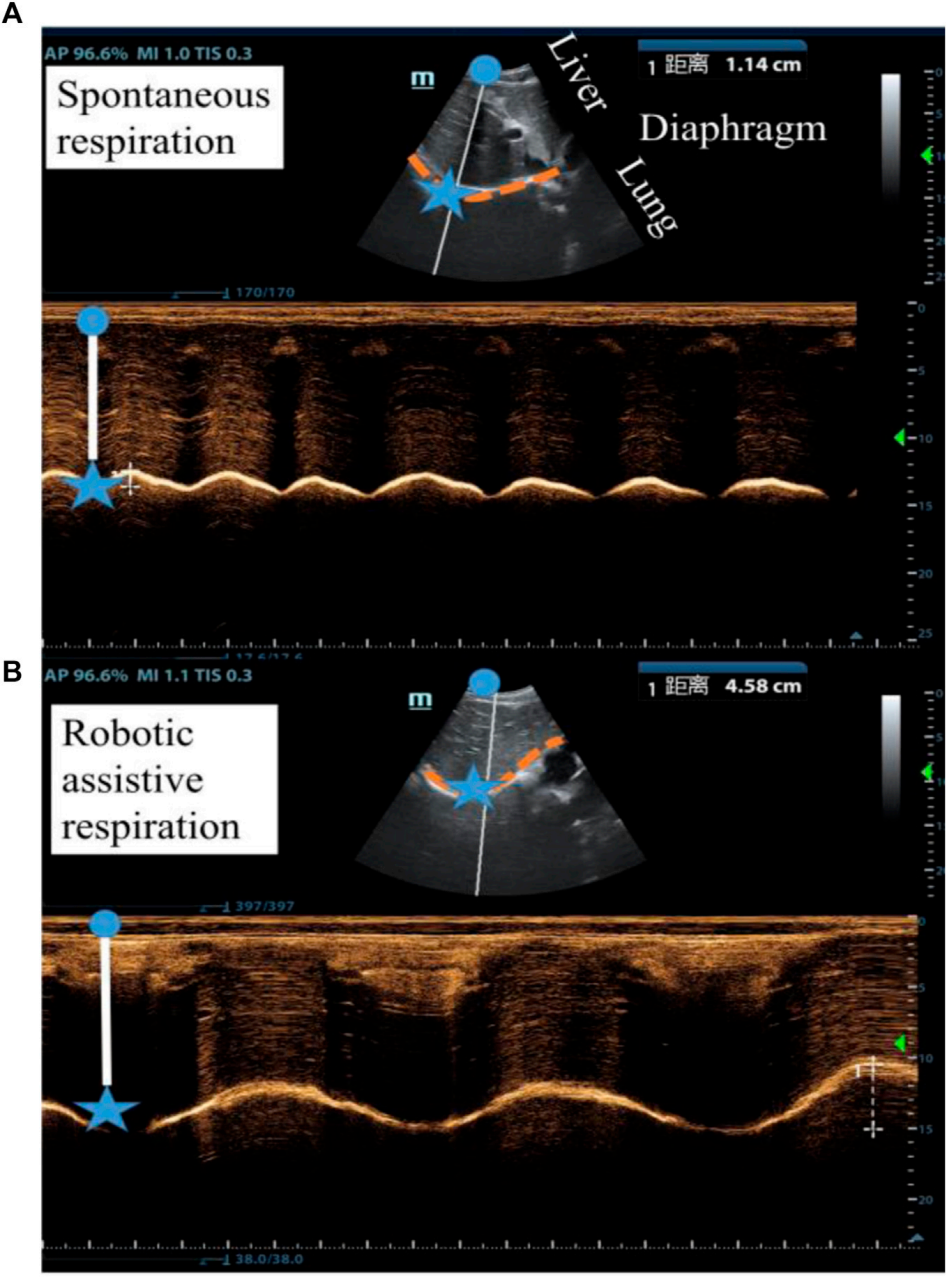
Ultrasound imaging of the diaphragm displacement (A) without robotic assistance and (B) with robotic assistance. (Participant 6).
